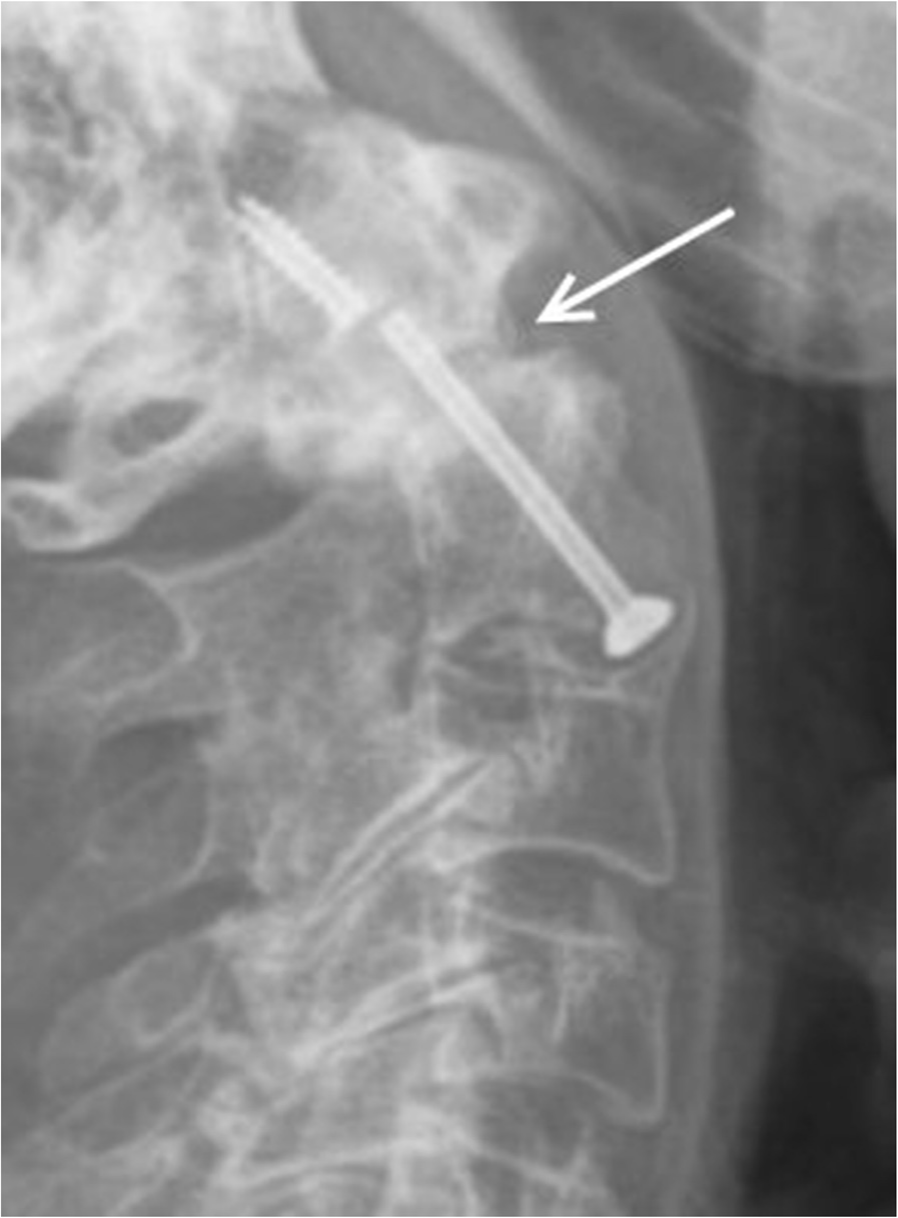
Lateral radiographs obtained 6 years after anterior screw fixation in a 77-year old man who suffered a type II odontoid fracture after fall of 3 meter. The image demonstrates a pseudarthrosis at the base of C2 with a breakage of the screw.
